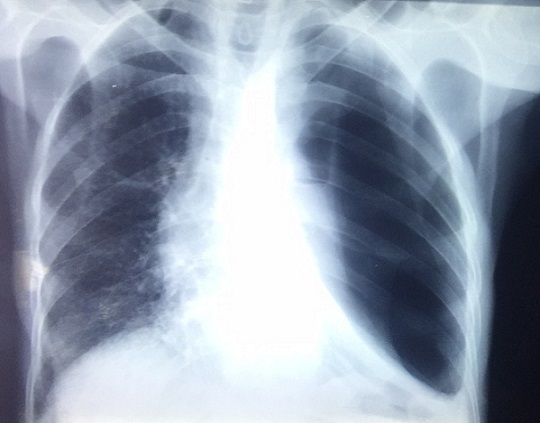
Radiographie thoracique montre une une hyperclarté de tout l’hémithorax gauche avec présence de deux images hydroaériques et une réaction pleurale avec refoulement des éléments du médiastin
